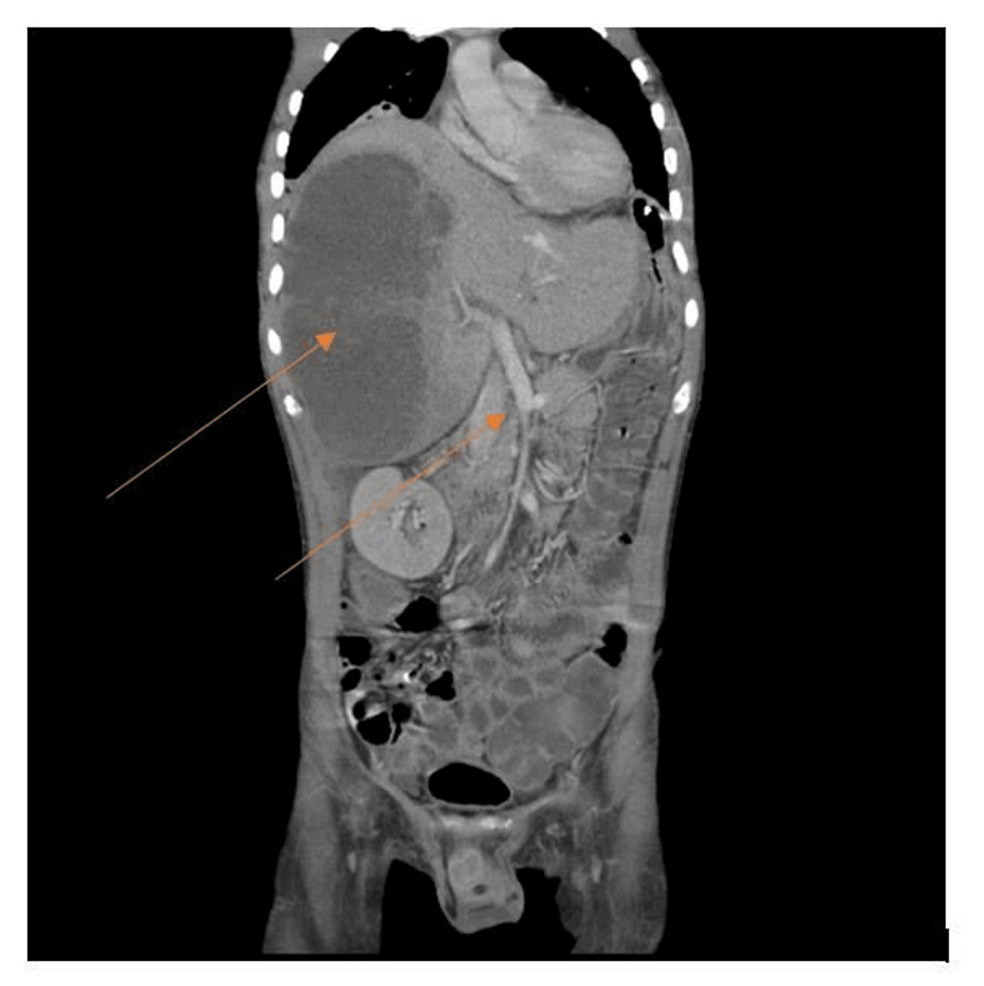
CT scan coronal view The image shows the enlarged liver compressing the gall bladder and right kidney, pushing it towards the psoas major muscle. The inferior vena cava has deviated from its normal course and appears to be compressing the portal vein and hepatic vein (indicated by the arrows) CT: computed tomography
